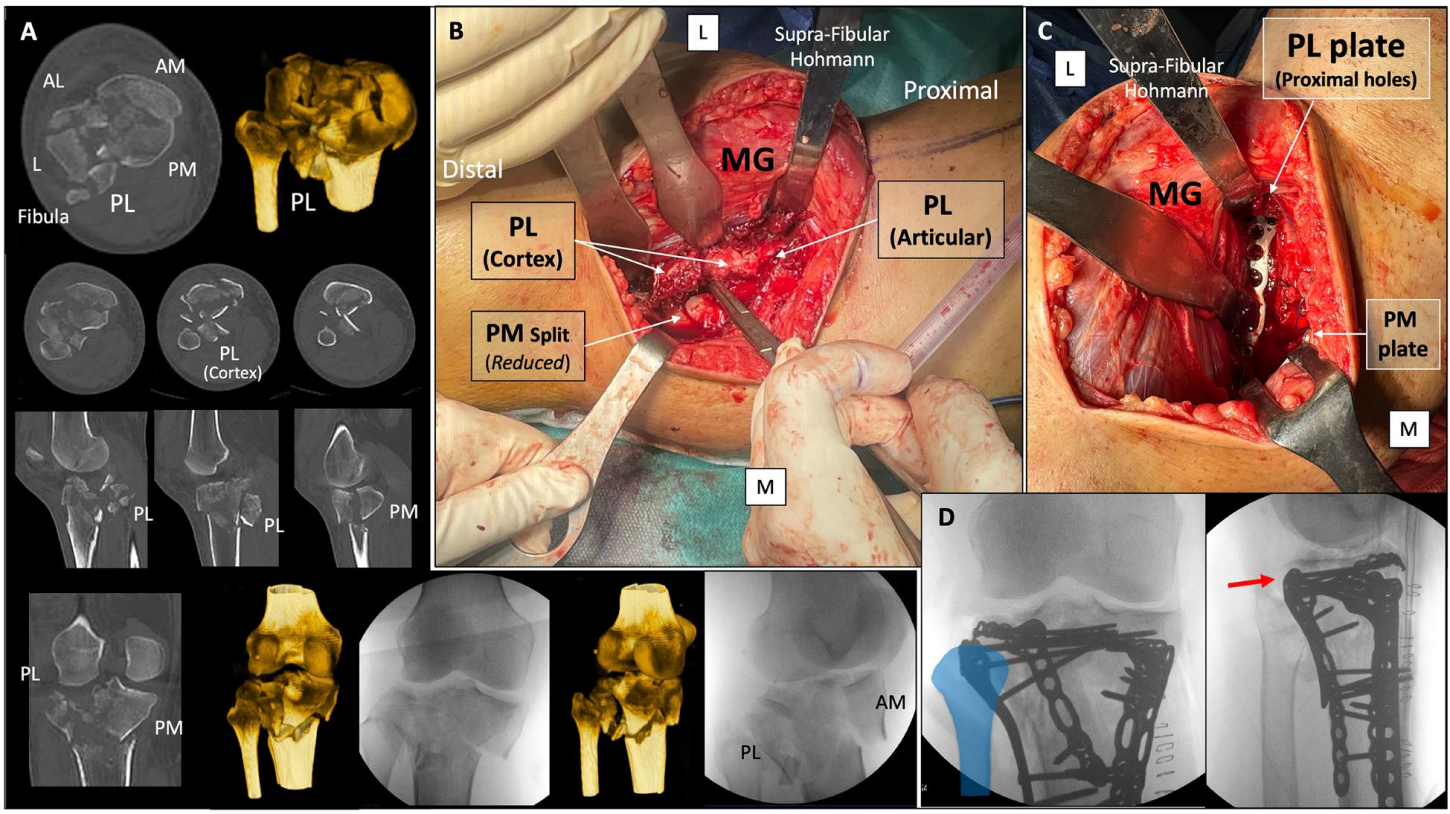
Clinical case example of PL column exposure and PL plate fixation via MOL approach in a complex multi-columnar fracture in a 43-year-old man with bulky gastrocnemius. A Pre-operative CT scan and fluoroscopy. Note the comminution and the displacement of the PL cortex fragments. B Exposure of the PL and PM columns using the MOL approach. Note the location of the supra-fibular Hohmann and that the full exposure of the PL column was achieved from the PL articular level (including the PL corner) to the most distal cortical fragments. PM column split is partially reduced in this image. C Surgical image of the PL plate location and the feasibility for proximal screw placement near to the supra-fibular Hohmann. The distal Hohmann rests on the PL plate to avoid unnecessary tension. PM plate is partially visible in this image. D Intraoperative fluoroscopy. Fibula marked in blue. Proximal screw of the PL plate (red arrow) was feasible with an appropriate direction through the MOL approach. In this case, simultaneous fracture control was performed in a fixed prone semi-lateral position, combining an anteromedial approach (flexing the knee for anteromedial reduction and plating), the pre-fibular window of the extended anterolateral approach (lateral plate and anterolateral horizontal plate) and the MOL approach (PM and PL plates)
